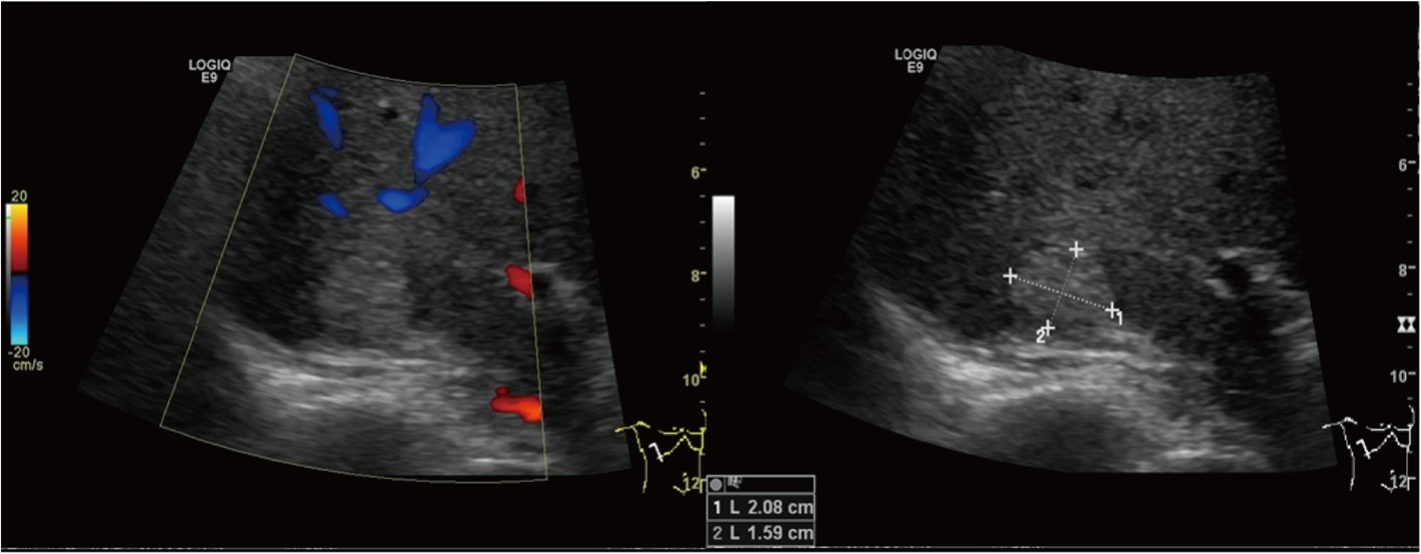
B-ultrasound reexamination showing a 2.1 cm × 1.6 cm echo node on the right lobe of the liver
